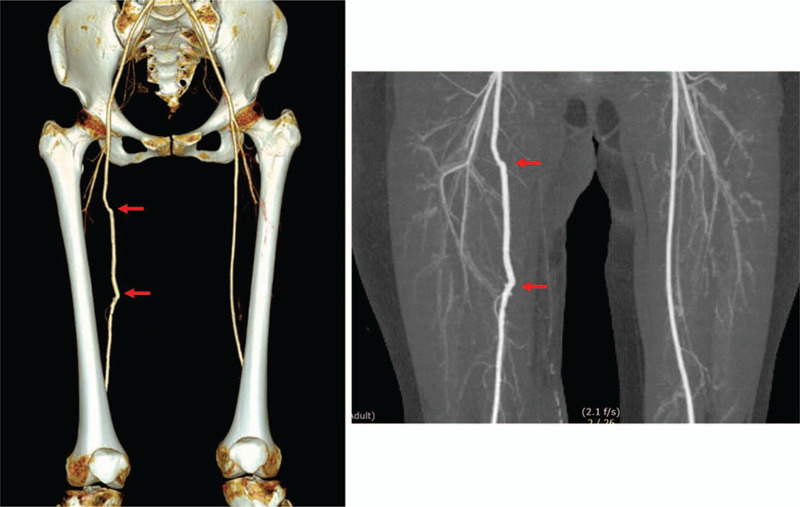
CT obtained at 12 months after excision of the mass and femoral-to-femoral graft interposition using the greater saphenous vein. Vessel reconstruction CT images confirmed normal continuous flow of the femoral artery.
